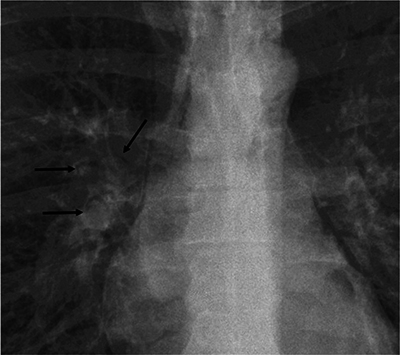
Right hilar air crescent on a chest radiograph (arrows).
